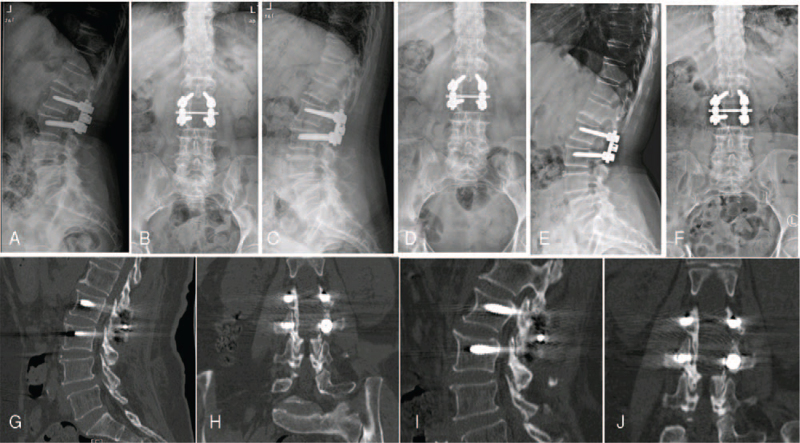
(A, B) were the anteroposterior and lateral radiographs of the lumbar spine on the 1 month after the operation, respectively, showed the internal fixation was in good position. Three months (C, D, G, H) and half a year (eE, F, I, J) after the operation, reexamination of lumbar anteroposterior and lateral radiographs and lumbar CT showed that the internal fixation device was in good position, and partial fusion of facet joint could be seen.
